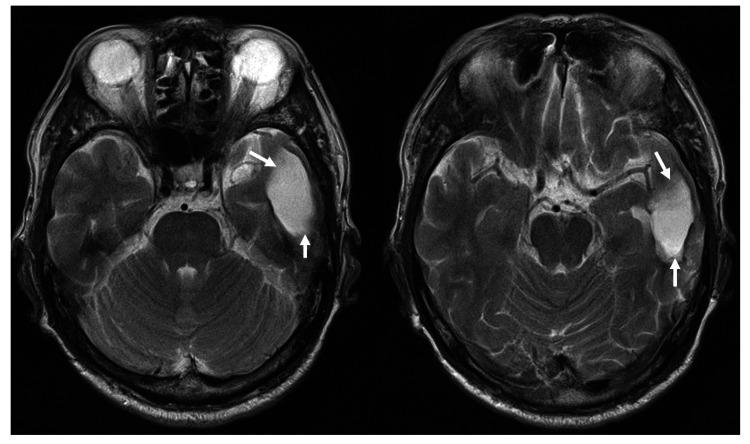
Magnetic resonance (MR) images seven months after the onset of intracerebral hemorrhage. No abnormal lesion signal around the hematoma’s cavity (arrows) were found on T2-weighted MR images.
